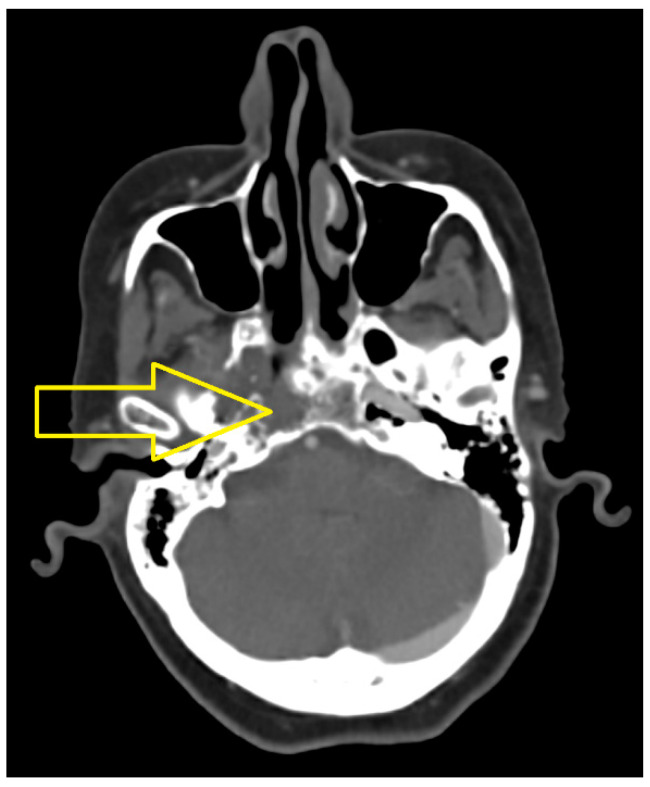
CT scan of the cervical region—December 2024. The focal lysis was maintained in the cavum (yellow arrow). No submandibular or latero-cervical lymphadenopathies.
